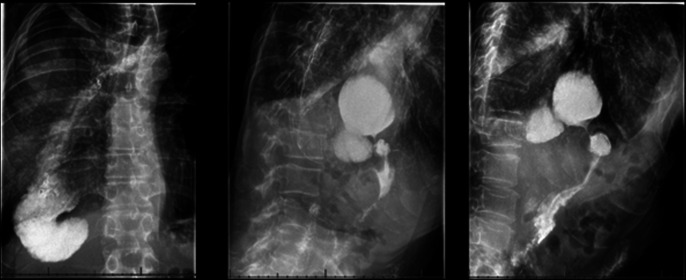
Images from X-ray barium swallow with contrast showing widely patent esophagogastric anastomosis, redundant intrathoracic portion of the proximal gastrointestinal tract, and a narrowed lumen at the level of the diaphragm.
